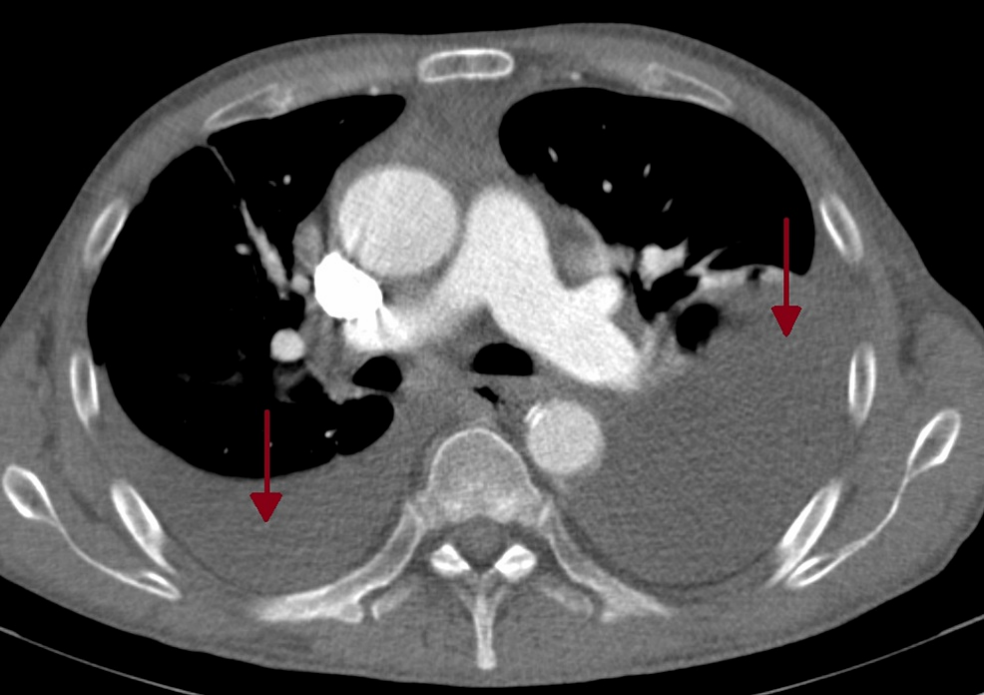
Chest computed tomography Red arrows: bilateral pleural effusion
